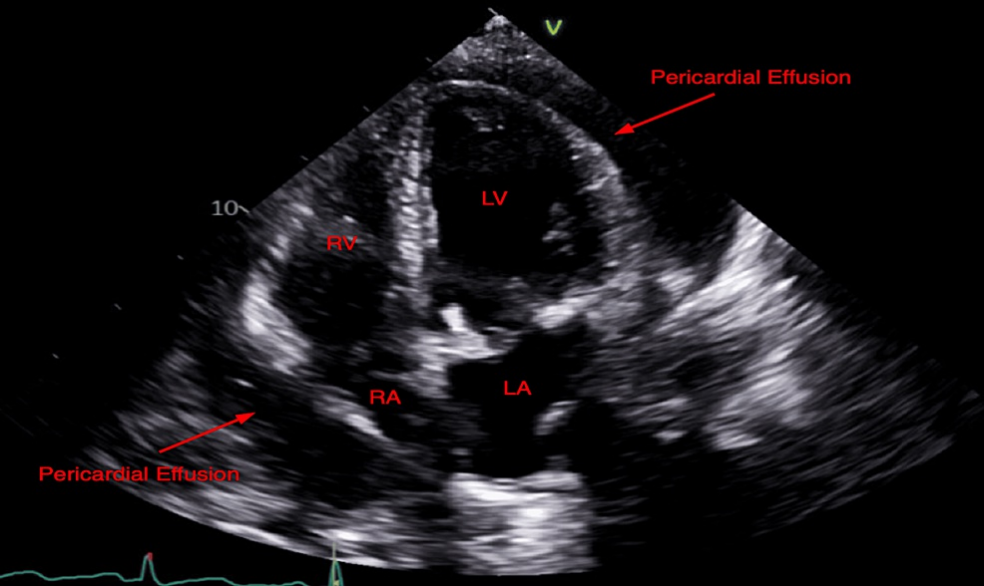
Apical four-chamber echocardiogram - Moderate-to-large circumferential pericardial effusion with right atrial invagination RA, right atrium; RV, right ventricle; LA, left atrium; LV, left ventricle.
